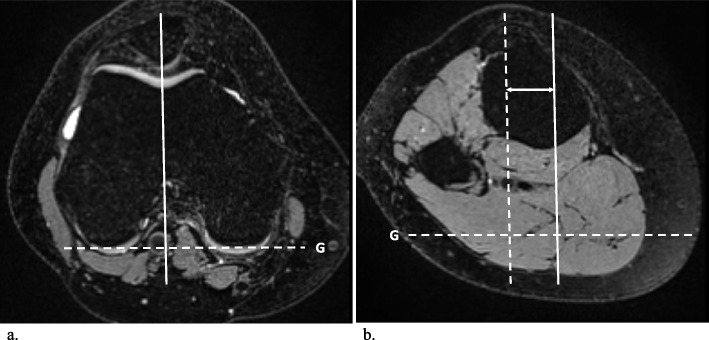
Measurements of the trochlear morphology shown on axial MR images of the right knee: the tibial tuberosity to trochlear groove distance is measured by calculating the transverse length between the trochlear groove (a) on the femur and tibial tuberosity (b) on axial images
